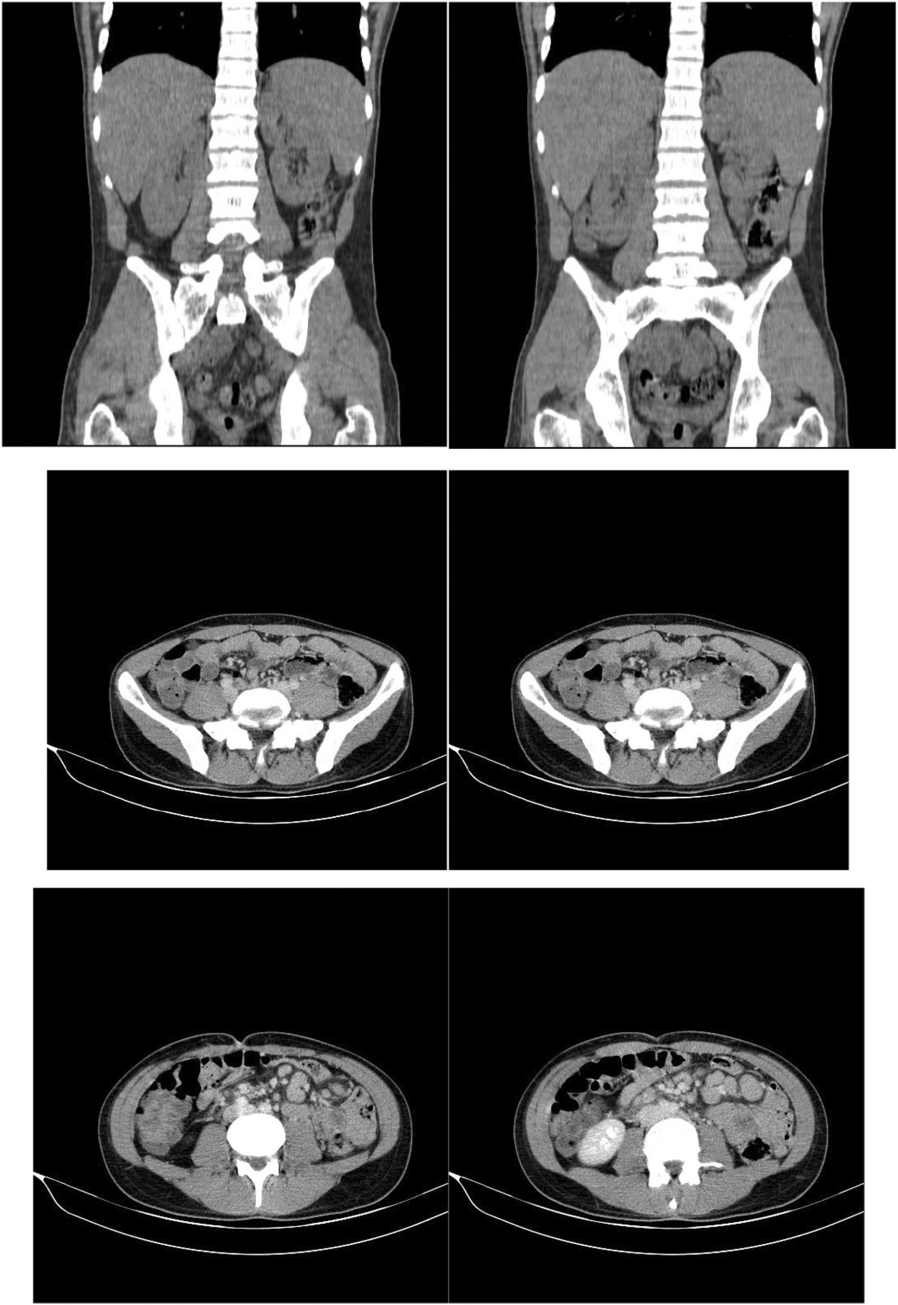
Abdomio‐pelvic CT scan. Mild splenomegaly (150 mm) and multiple mesenteric lymphadenopathies, with a conglomeration pattern and maximum size of 17 mm were detected.
